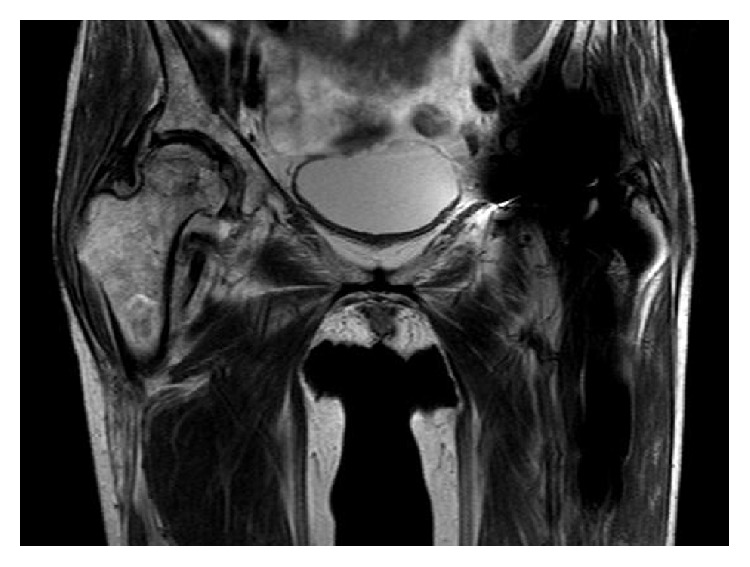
T1W mid-coronal section MRI showing osteonecrosis of the right femoral head.
